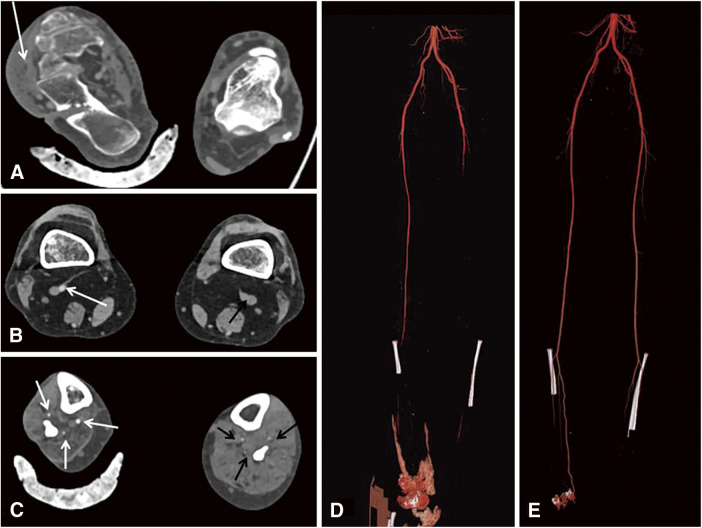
Fig. 1 (A) Axial CT image of the lower limbs at the level of the ankle shows diffuse subcutaneous edema involving the right lower leg and foot (white arrow). (B) Arterial phase CT angiography image shows luminal enhancement of the right popliteal artery (white arrow). There is no corresponding luminal enhancement of the left popliteal artery (black arrow). (C) The venous phase image shows good luminal enhancement of the right anterior tibial artery, posterior tibial artery, and peroneal trunk (white arrows). On the left side, faint luminal enhancement of the anterior tibial artery, posterior tibial artery, and peroneal trunk is noted (black arrows). (D) 3D reconstruction of CT angiography run in the arterial phase shows a considerable lag of luminal enhancement of left lower limb arteries as compared to the right side. (E) 3D reconstruction of the venous phase run shows further distal luminal enhancement in the left lower limb arteries, however, still lags behind the right lower limb. CT: computed tomography
